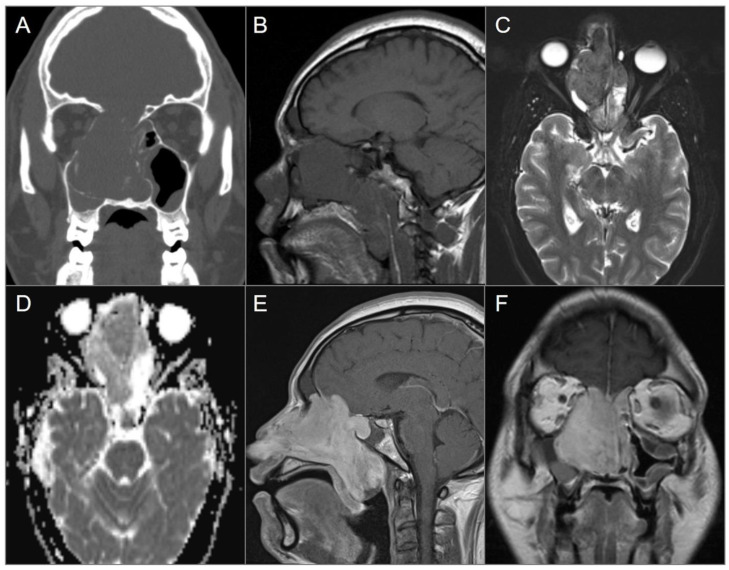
Nasal osteosarcoma invading skull base. (A) Coronal CT image shows a bulky expansile soft tissue density lesion in the nasal cavity, eroding the anterior skull base, nasal septum, and right lamina papyracea. (B) Sagittal T1W and (C) axial fat-saturated T2W MR images reveal slightly hypointense signal of the lesion on both sequences as well as mucus accumulation in the adjacent paranasal sinuses due to obstruction of the drainage pathways. (D) ADC map reveals mild diffusion restriction in the lesion anteriorly. (E) Sagittal and (F) coronal postcontrast T1W images demonstrate avid enhancement of the lesion with extension to the anterior cranial fossa, nasopharynx, and oropharynx.
